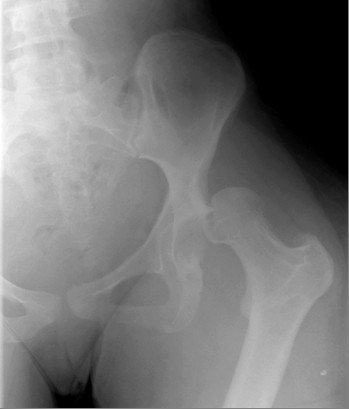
Radiograph of dislocated left hip.
